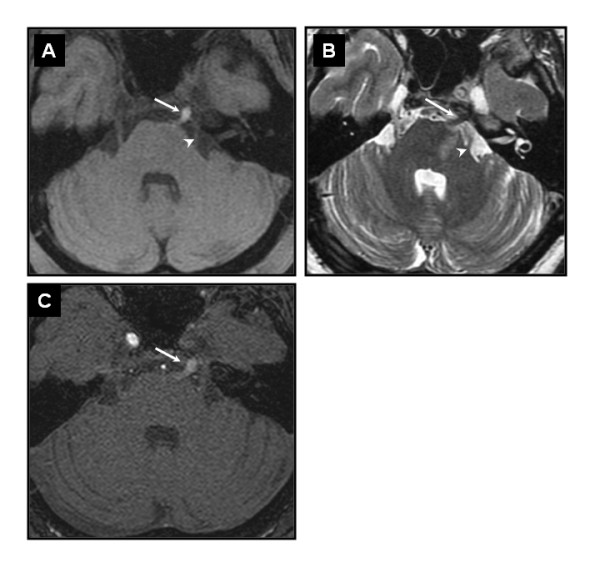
Thin axial sections MRI. Axial T1WI with fat-saturation (A), T2WI (B) and axial view of 3D-TOF MRA (C) showing the thrombus in the persistent PTA (*arrow*) appearing hyperintense in T1WI, hypointense on T2WI, medial to the cisternal portion of the trigeminal nerve (*arrowhead*).
